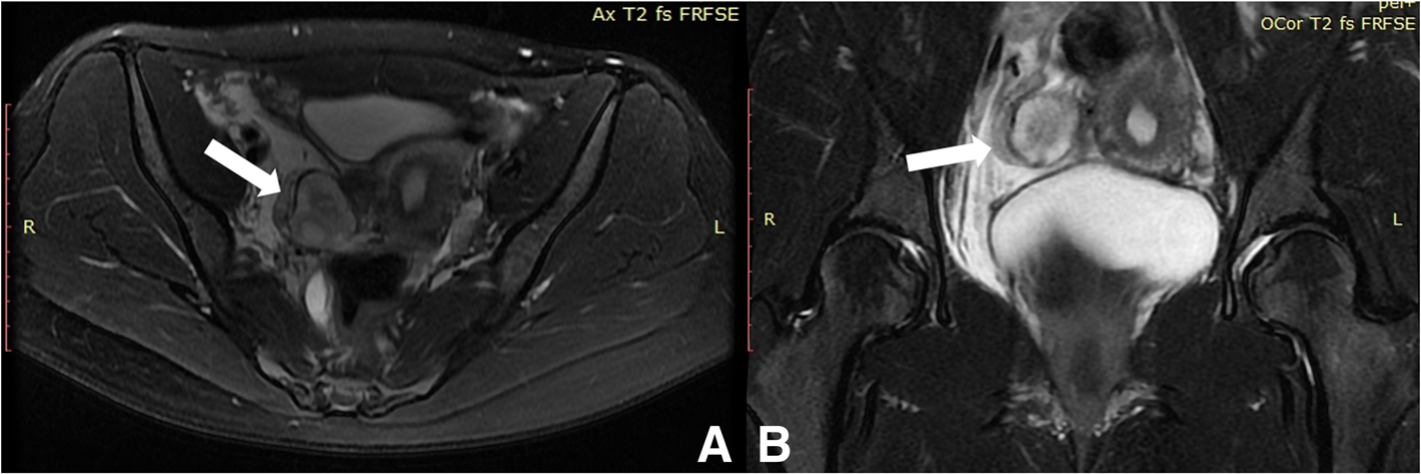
Pelvic magnetic resonance imaging: typical pelvic fibroma (white arrow) presenting as a well-delineated mass on the T2-weighted image. a T2-weighted image, transverse section; b T2-weighted image, coronal section
